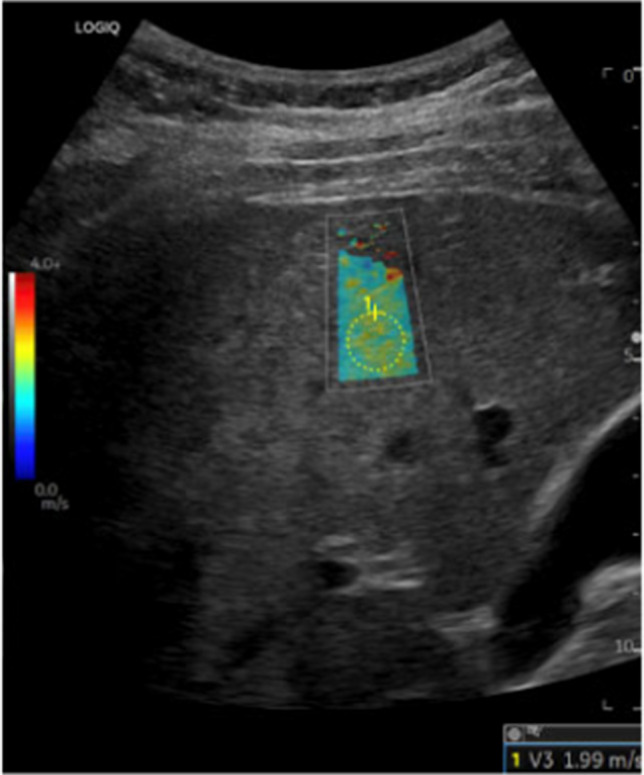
Transverse ultrasound elastography image of the right lobe of the liver in a 20-year-old male patient who is status post Fontan palliation. The image shows mild coarsening of the hepatic parenchymal architecture with an elastography shear wave speed of 1.99 m/s. The median shear wave velocity for this patient was 1.9 m/s, which is elevated
